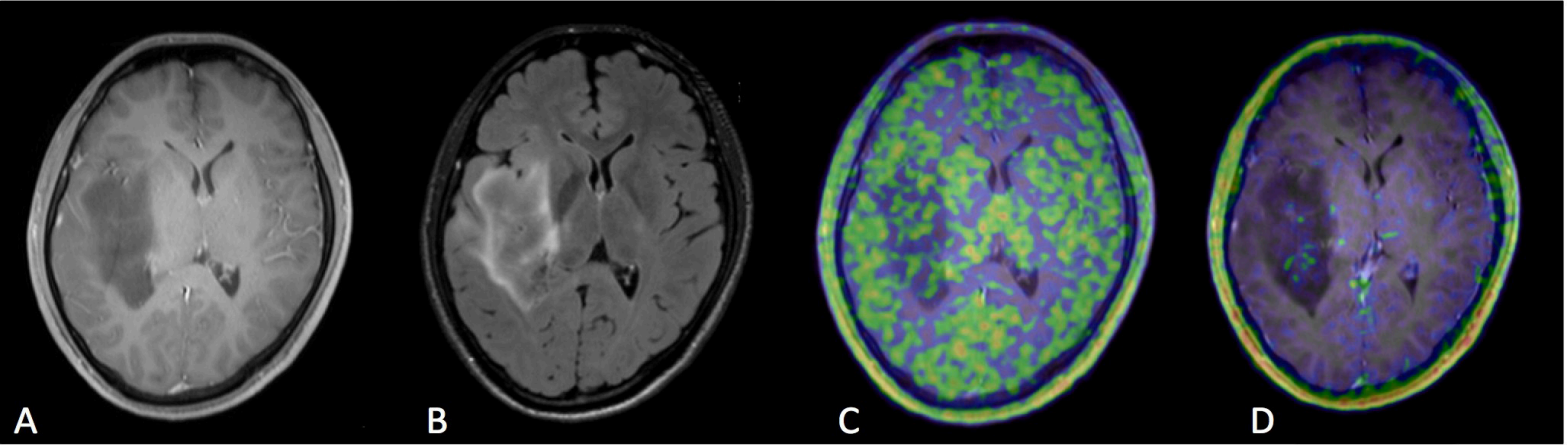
A: Gd contrast-enhanced T1-weighted image B: FLAIR (fluid-attenuated inversion recovery) image C: [^11^C]methionine positron emission tomography (MET-PET) D: anti- [^18^F]FACBC positron emission tomography (FACBC-PET) In a 21-year-old man, the lesion was confirmed as a diffuse low signal lesion without enhancement in Gd T1-weighted image and a slight high irregular lesion in FLAIR image on the left insula lobe. The lesion was diffuse astrocytoma, grade II, IDH mutant. MET-PET showed low uptake in the lesion as well as background. On the other hand, FACBC-PET showed low uptake in the lesion, but slight accumulation was found in small part of the tumor.
